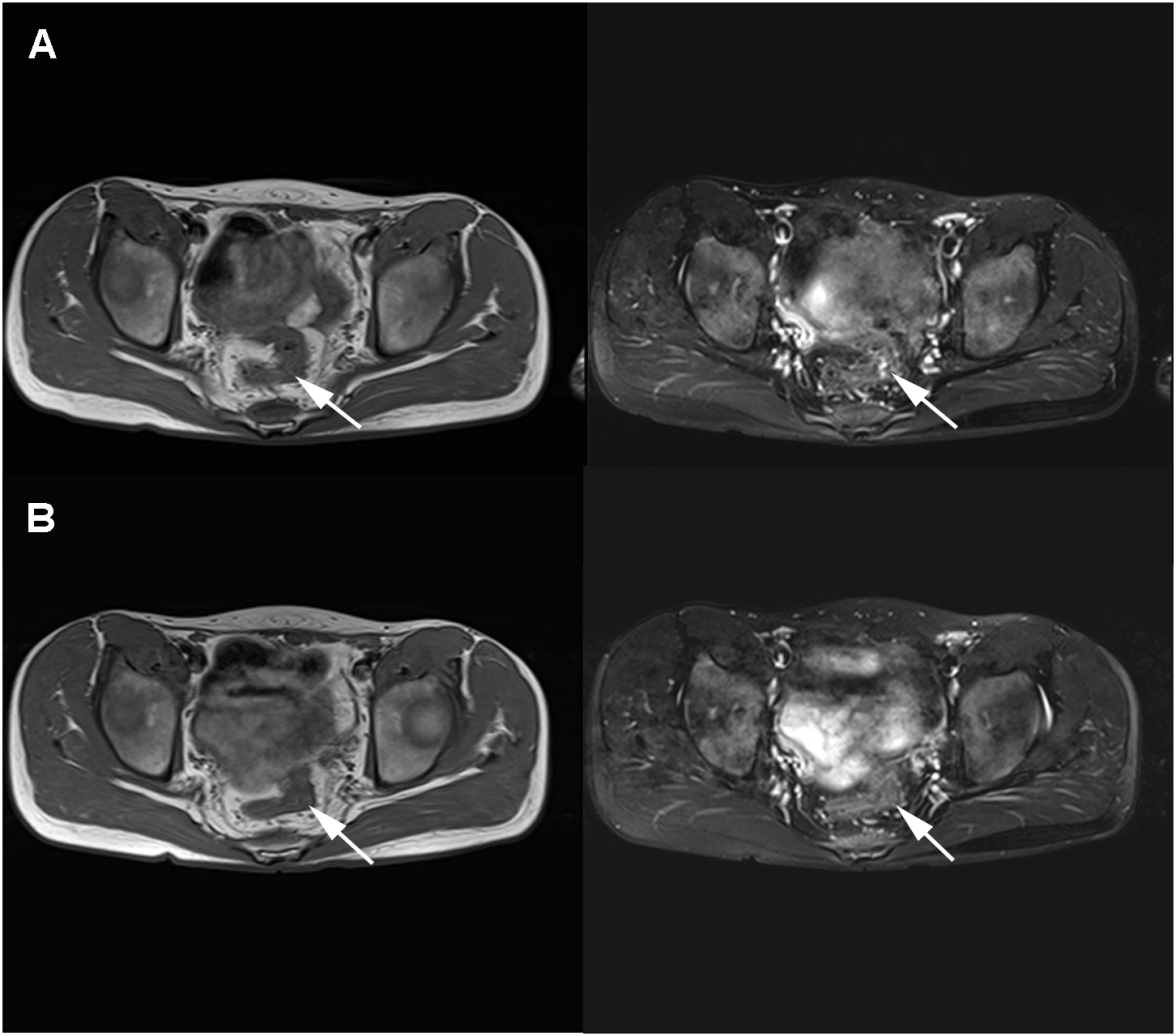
MRI review in March 2021 after neoadjuvant immunotherapy combined with chemotherapy showed a significantly smaller lesion than before (A). MRI review in April 2021 showed a further smaller lesion than before with no significant thickening (B).
